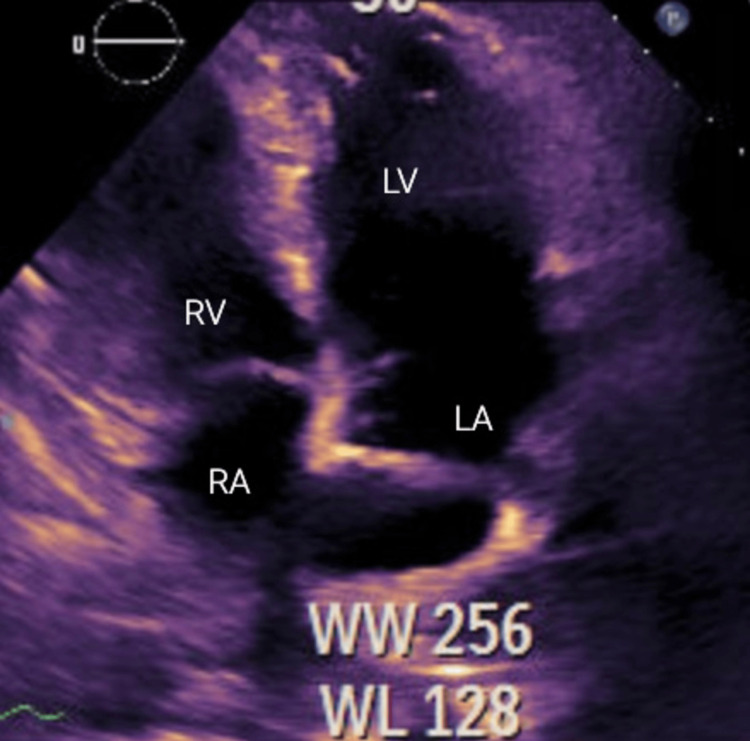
2D echocardiogram view post thrombolytic therapy showing the resolution of clot RA, right atrium; LA, left atrium; RV, right ventricle; LV, left ventricle
